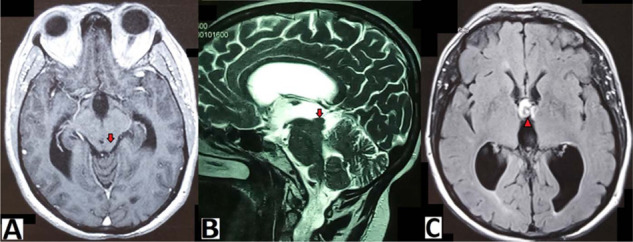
15 months postoperative MRI. (A) Axially injected tbl1 WI, (B) sagittal tbl2 WI, (C) axial FLAIR. The tectum lesion disappeared completely (arrow), with appearance of probable new location in the hypothalamus region (arrow head) and stabilization of the hydrocephalus.
